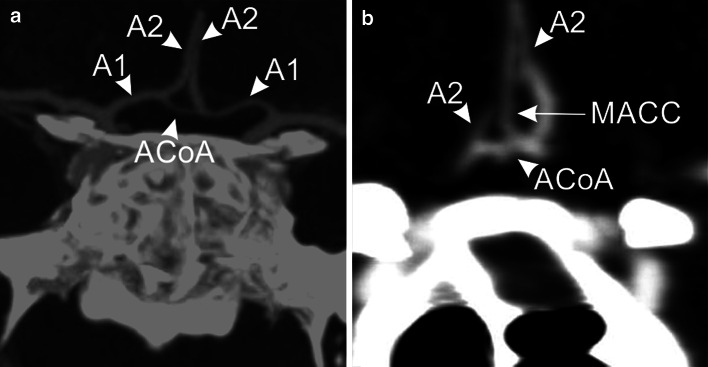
CTA images in transverse plane showing: a multi planar reconstruction image typical variant of the ACoA complex (type 1); b maximum intensity projection image presence of a third *A*2 segment of the anterior cerebral artery (*MACC* median artery of corpus callosum) (type 7). *A*1 A1 segment of the anterior cerebral artery; *A*2 A2 segment of the anterior cerebral artery; *ACoA* anterior communicating artery
